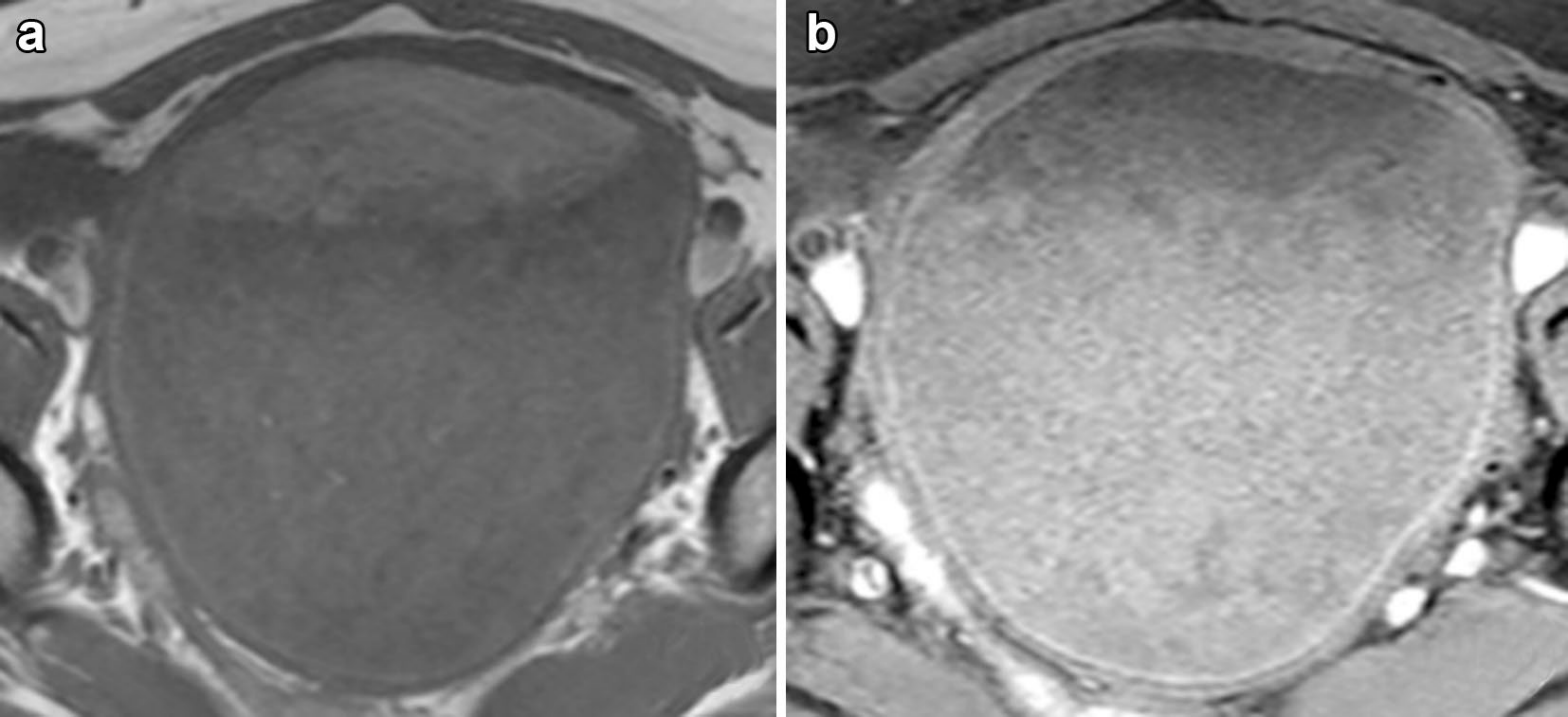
Magnetic resonance images. a A T1-weighted image shows a cystic tumor containing a fat–fluid level. b A fat-suppression image reveals fat suppression on the abdominal side of the niveau
